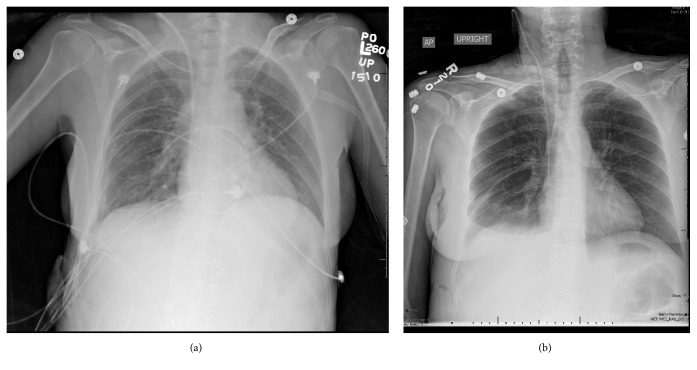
Chest x-rays with chest tube in situ and after removal of chest tube.
